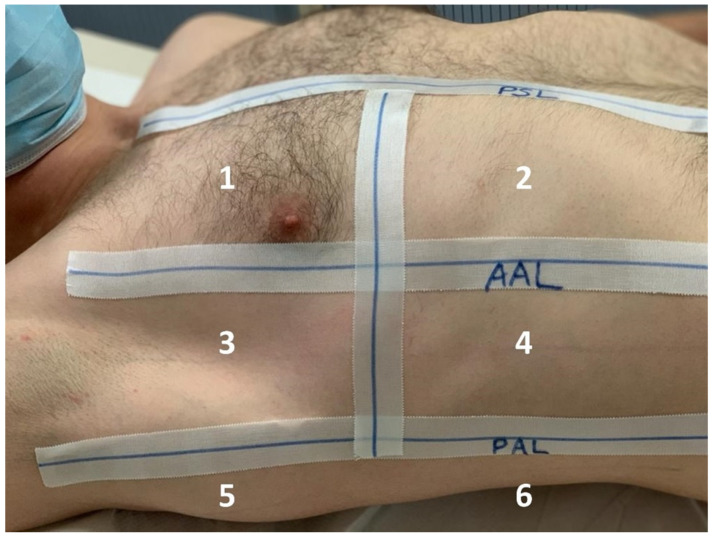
Lung areas for the right lung (labeled 1–6), PSL = parasternal line; AAL/PAL = anterior/posterior axillary line.
